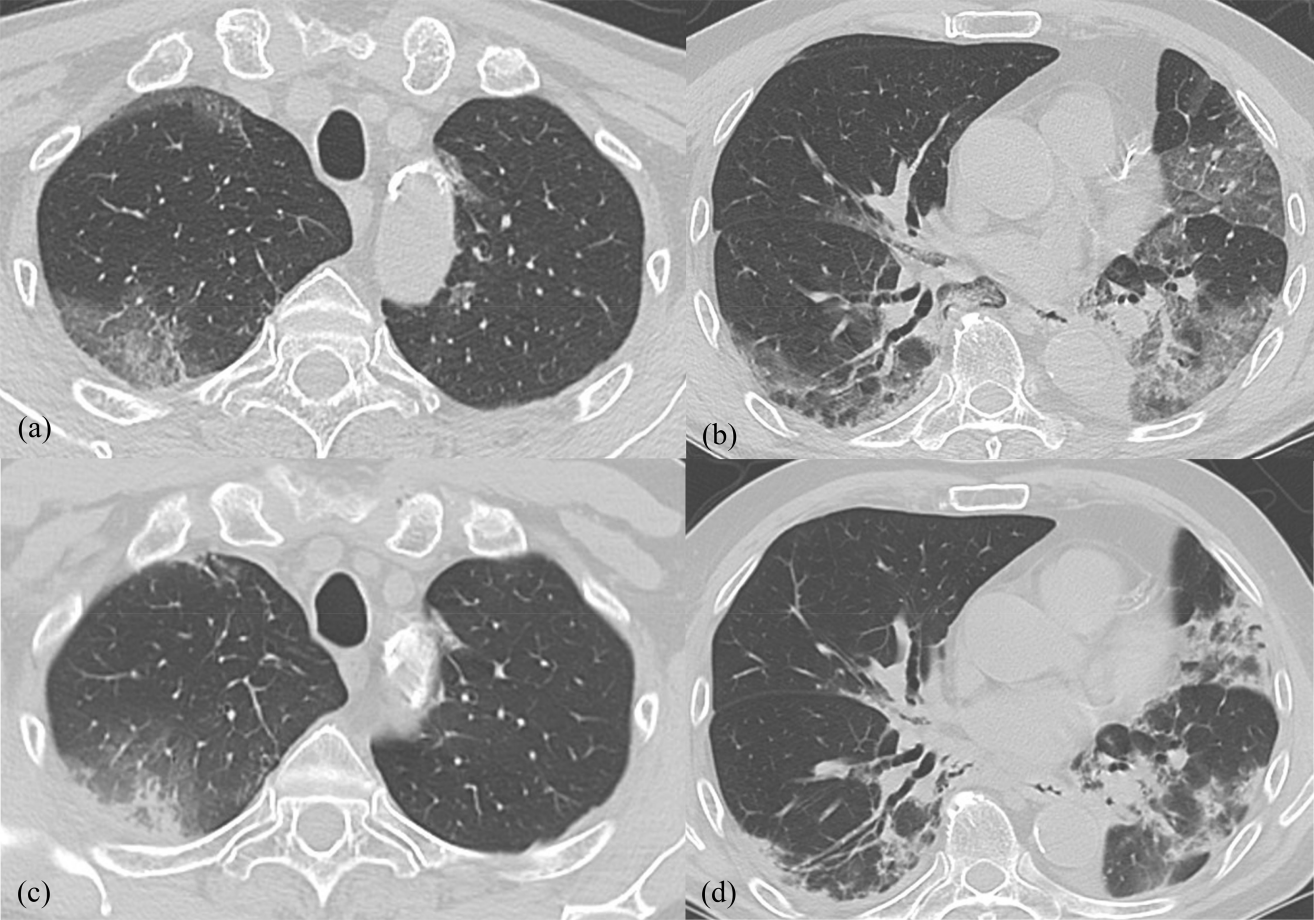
(a, b) High‐resolution chest tomography (HRCT) image at 3 months after starting regorafenib. Ground‐glass opacity (GGO) appeared in both lung fields. (c, d) HRCT image at 1 week after GGO appearance. Patchy infiltration shadow and consolidation replaced GGO in both lung fields.
